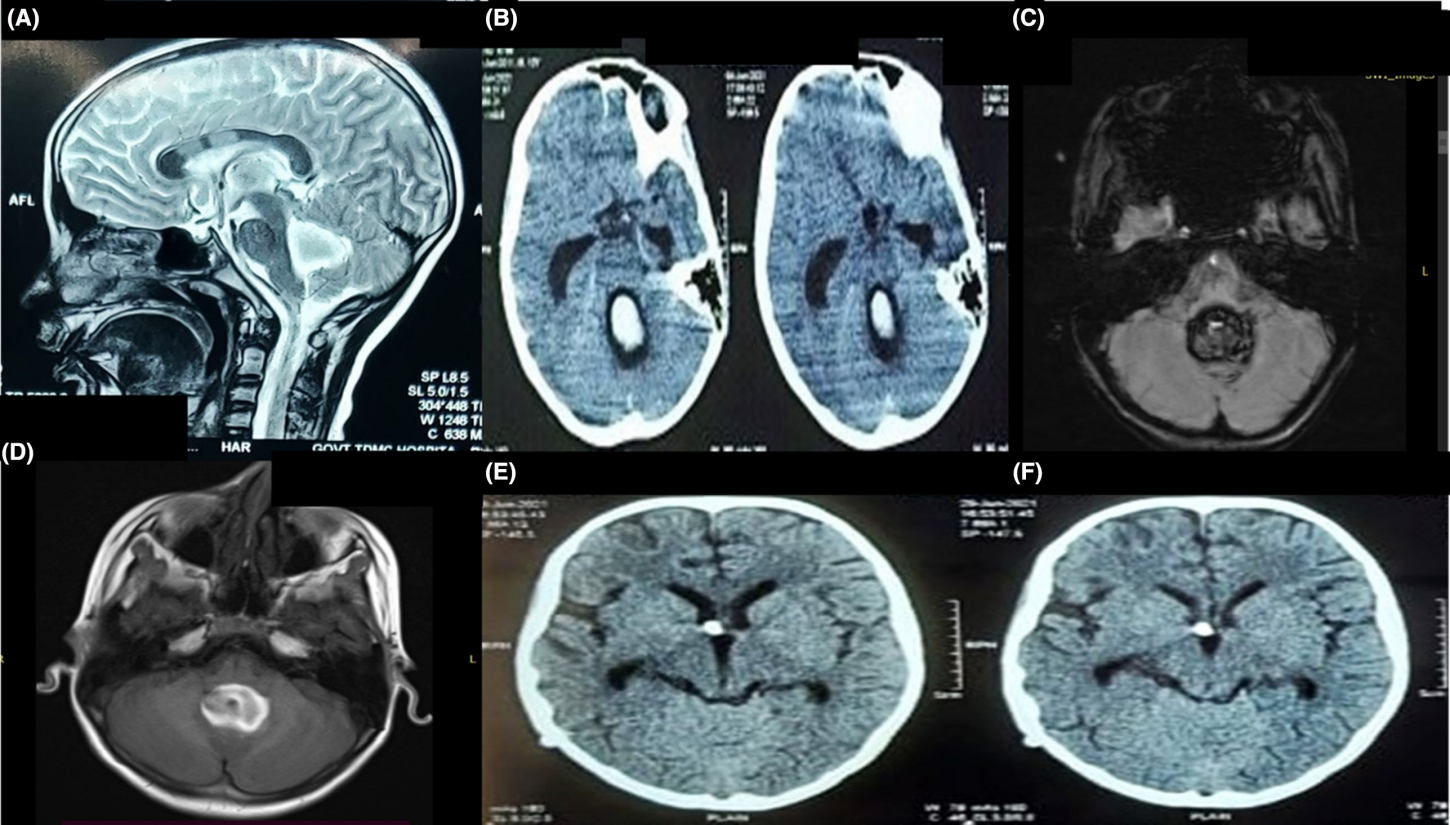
(A) Magnetic resonance imaging (MRI) showing isolated hemorrhage in the fourth ventricle. (B) CT scan showing a hyperdense area of blood density noted within the fourth ventricle, likely intraventricular hemorrhage. Upstream dilatation of bilateral lateral and third ventricle is noted, possibly due to the obstructive hydrocephalus. (C) Susceptibility weighted image showing blooming foci in the fourth ventricle. (D) T1‐weighted image showing hyperintense area within the fourth ventricle. (E, F) CT scan taken after ventriculoperitoneal shunt showing EVD tube in situ with tip in the right lateral ventricle. Mild hydrocephalus (reduced) seen.
